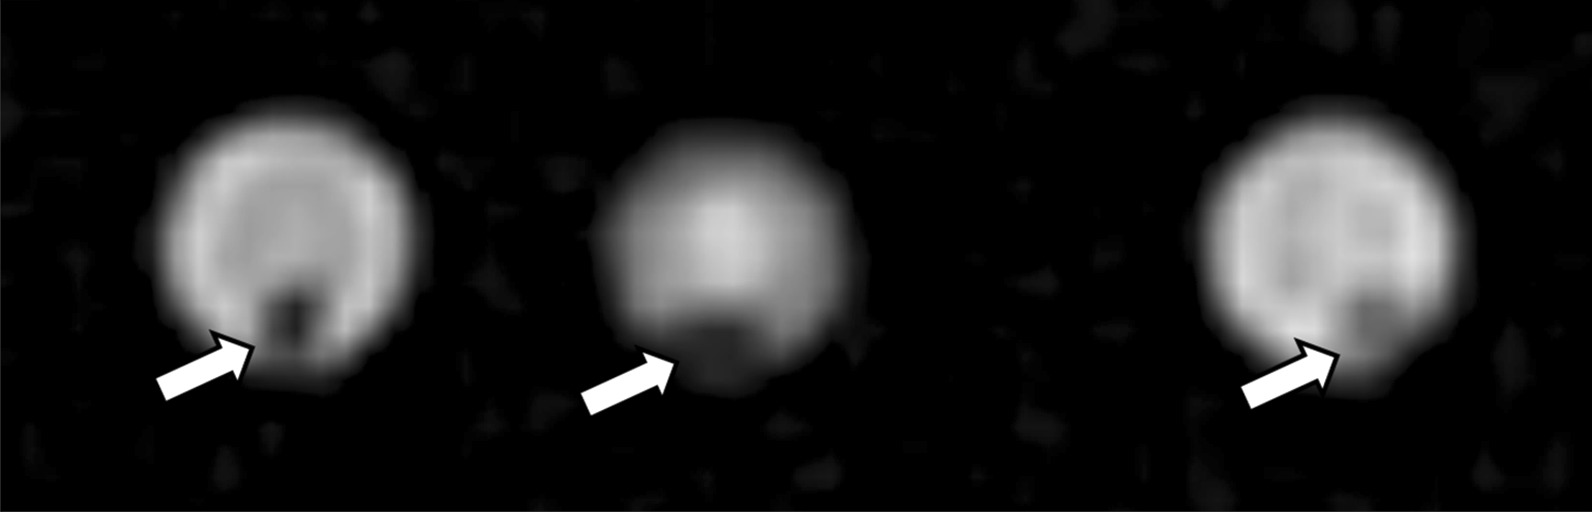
In vitro MRI of xanthine urinary stones: axial UTE-weighted MR image shows stones (arrows) as hypointense signal voids within hyperintense saline-filled syringes
